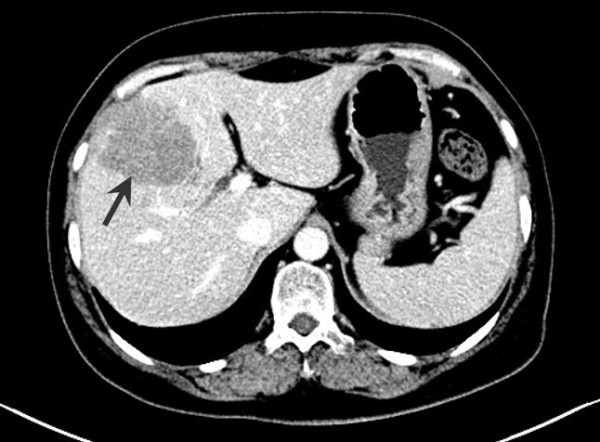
Contrast-enhanced CT axial section of the abdomen showing ahypodense lesion in the right lobe of the liver. The lesion is differentiated from the surrounding hepatic tissue by lower enhancement.
